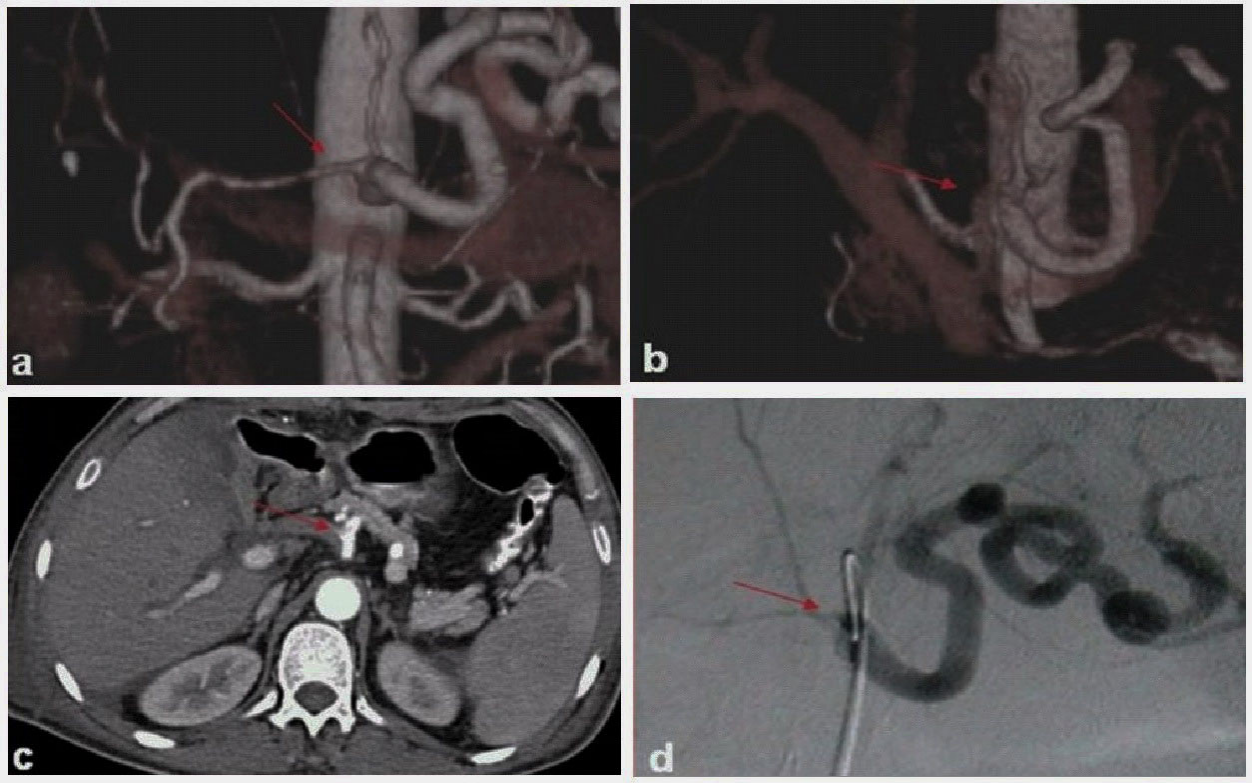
An occlusion at the common hepatic artery. (a) Preocclusion VRT image demonstrates an irregular and diffuse low-grade stenosis (arrow). Follow-up examination 2 weeks later demonstrates occlusion in (b) VRT, (c) axial CT, and (d) DSA images.
